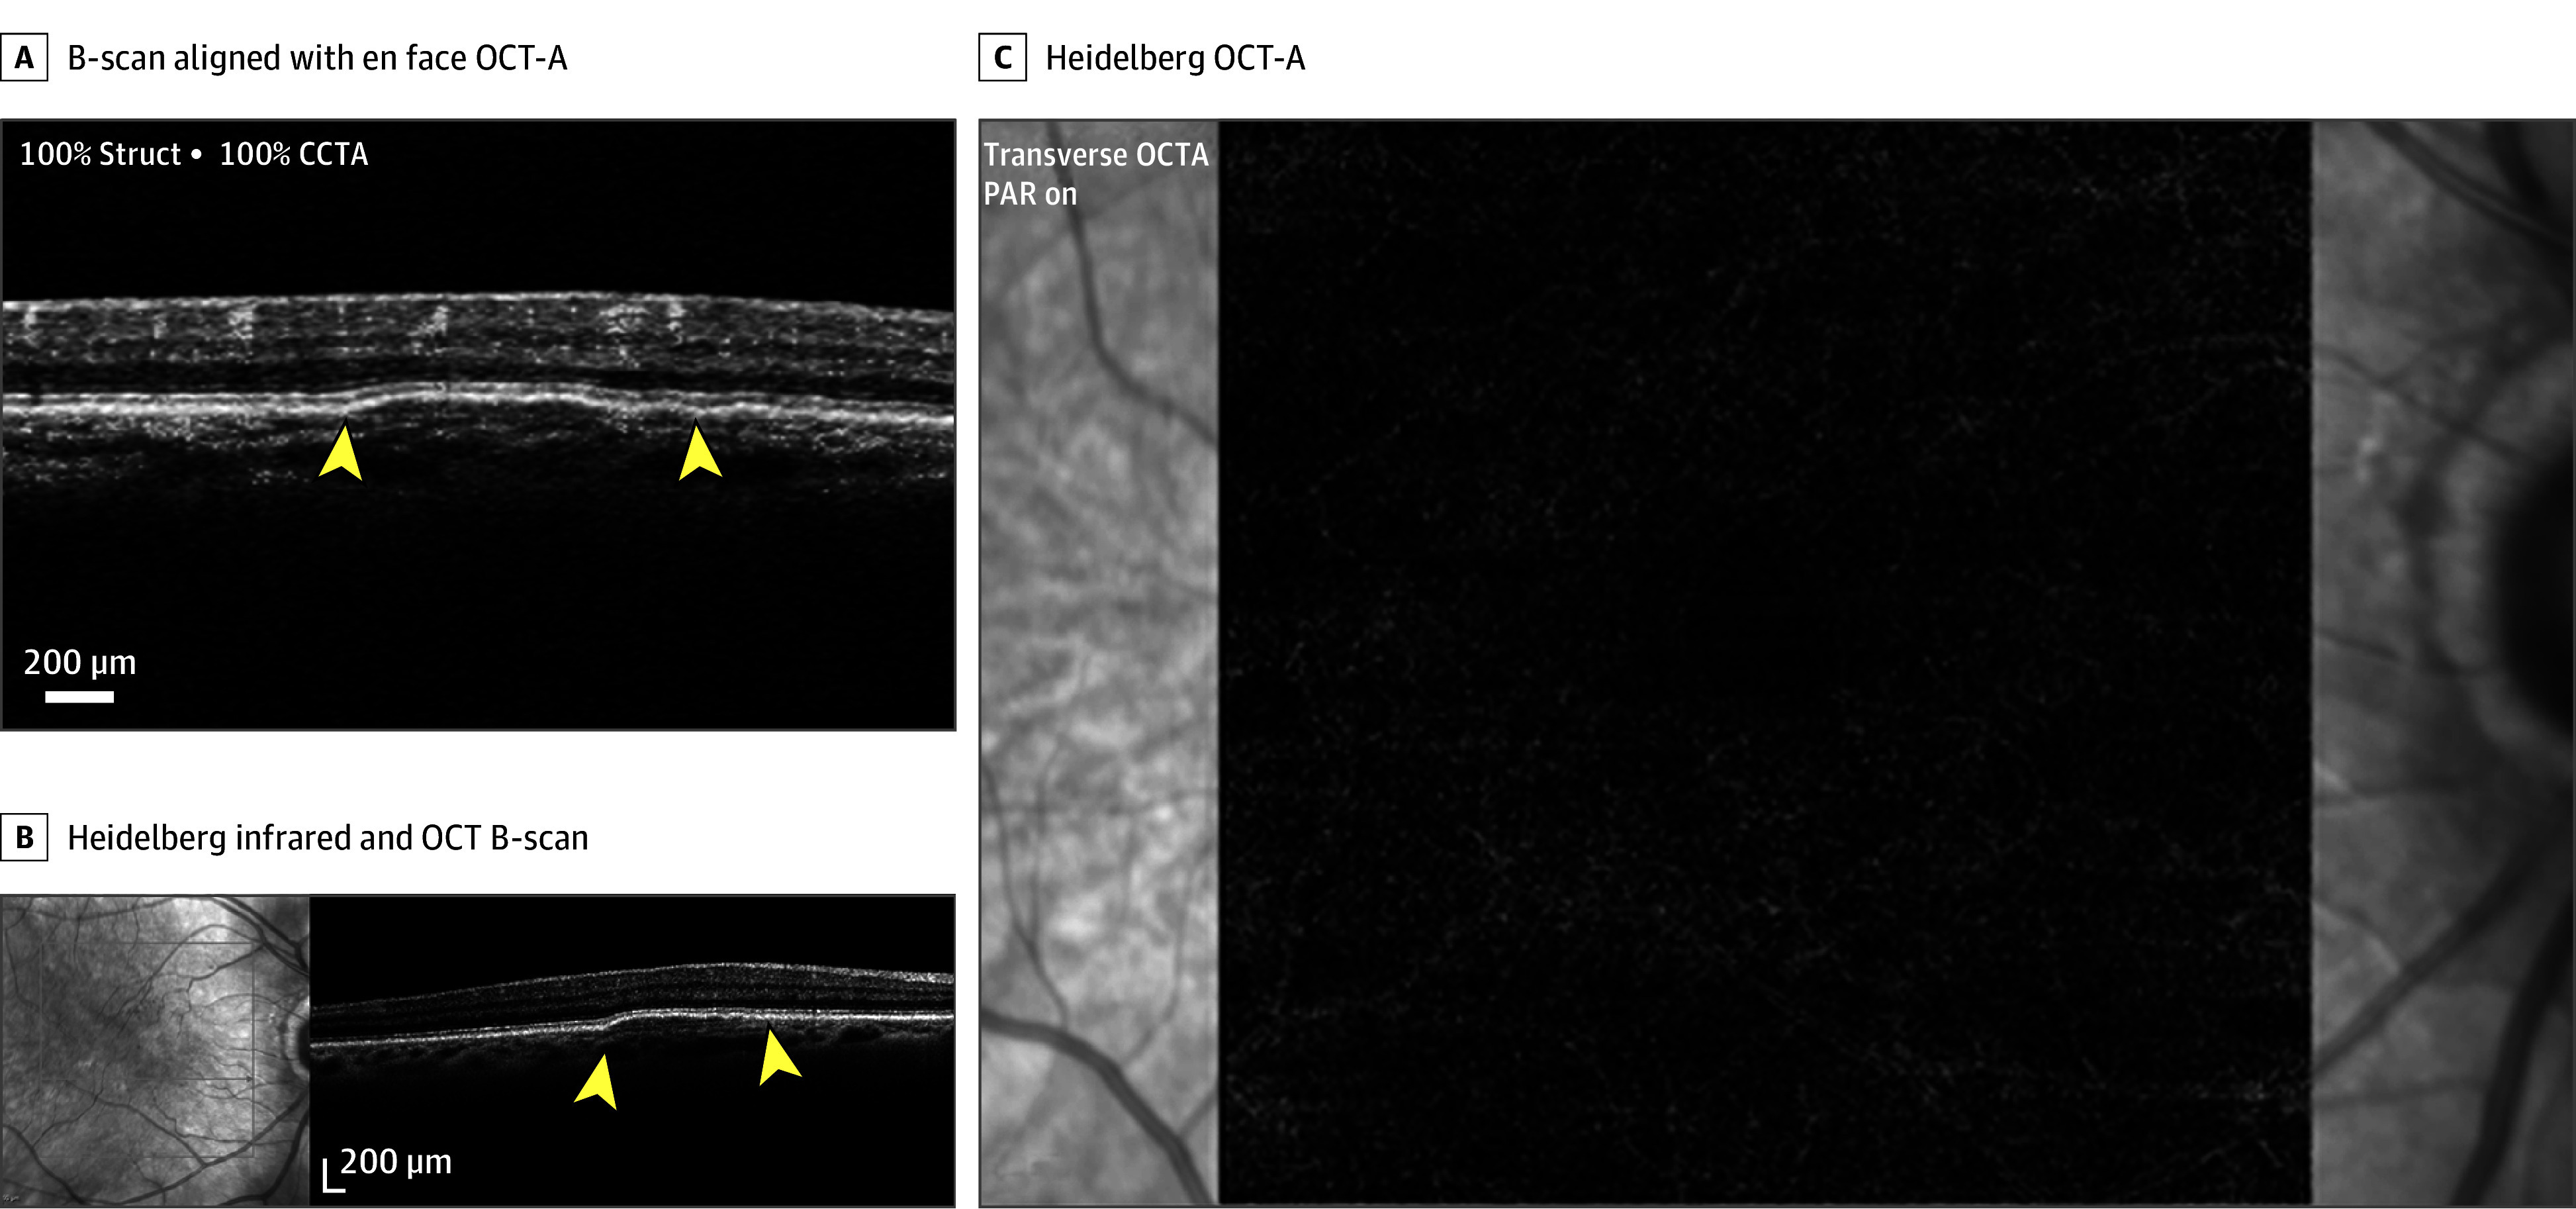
Image Showing a Thick Double-Layer Sign That Does Not Contain a Nonexudative Neovascular Membrane A, The brightness scan (B-scan) that aligns with the enface optical coherence tomography angiography (OCT-A) image and the boundary is indicated by yellow arrowheads. B, Heidelberg OCT-A shows the absence of nonexudative neovascular membrane. C, Heidelberg infrared and OCT B-scan shows the double-layer sign and the boundary is indicated by yellow arrowheads. CCTA indicates coronary computed tomography angiography; PAR, persistent avascular retina.
